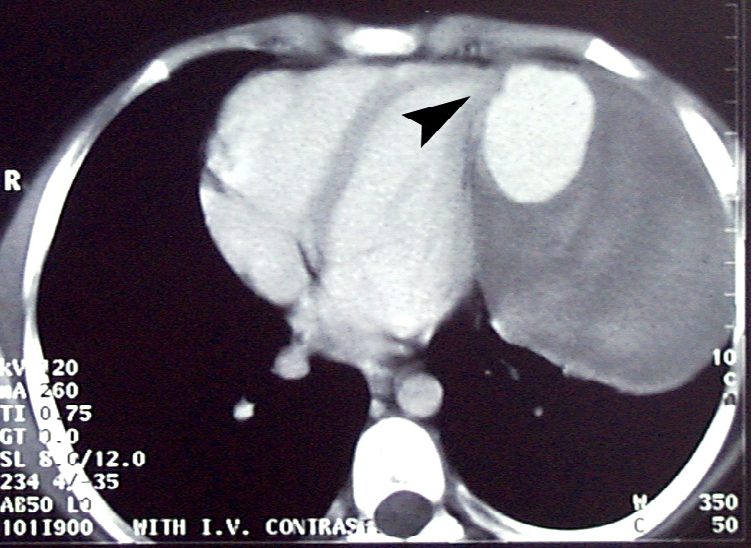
Chest computed tomography scan with contrast at T7 level. Arrowhead marks the ostium.
